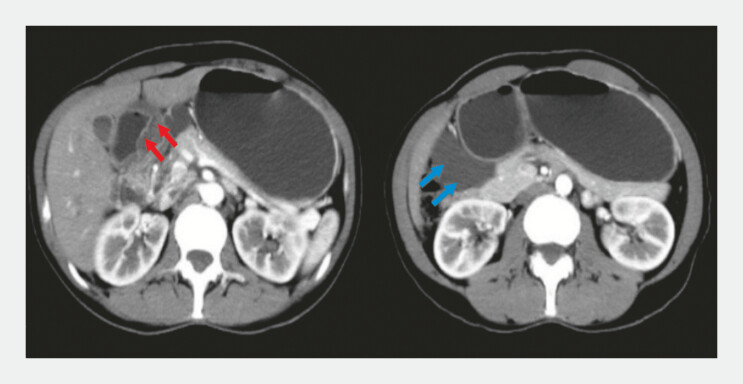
Full abdominal computed tomography with contrast enhancement. Diffuse thickening of the gastric antrum wall (red arrow). Hypodense perigastric focus (blue arrow).
